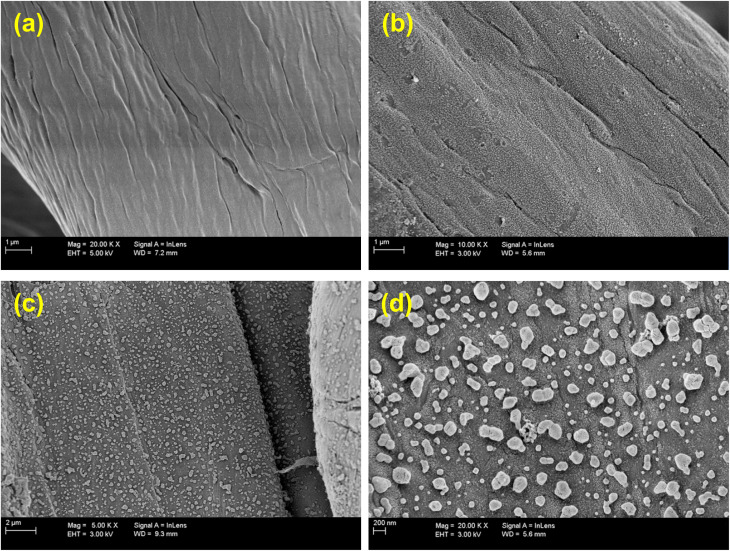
FE-SEM images of pristine CT (a), TiO_2_/CT (b), and Ag-Ag_2_S/TiO_2_/CT (c and d).
